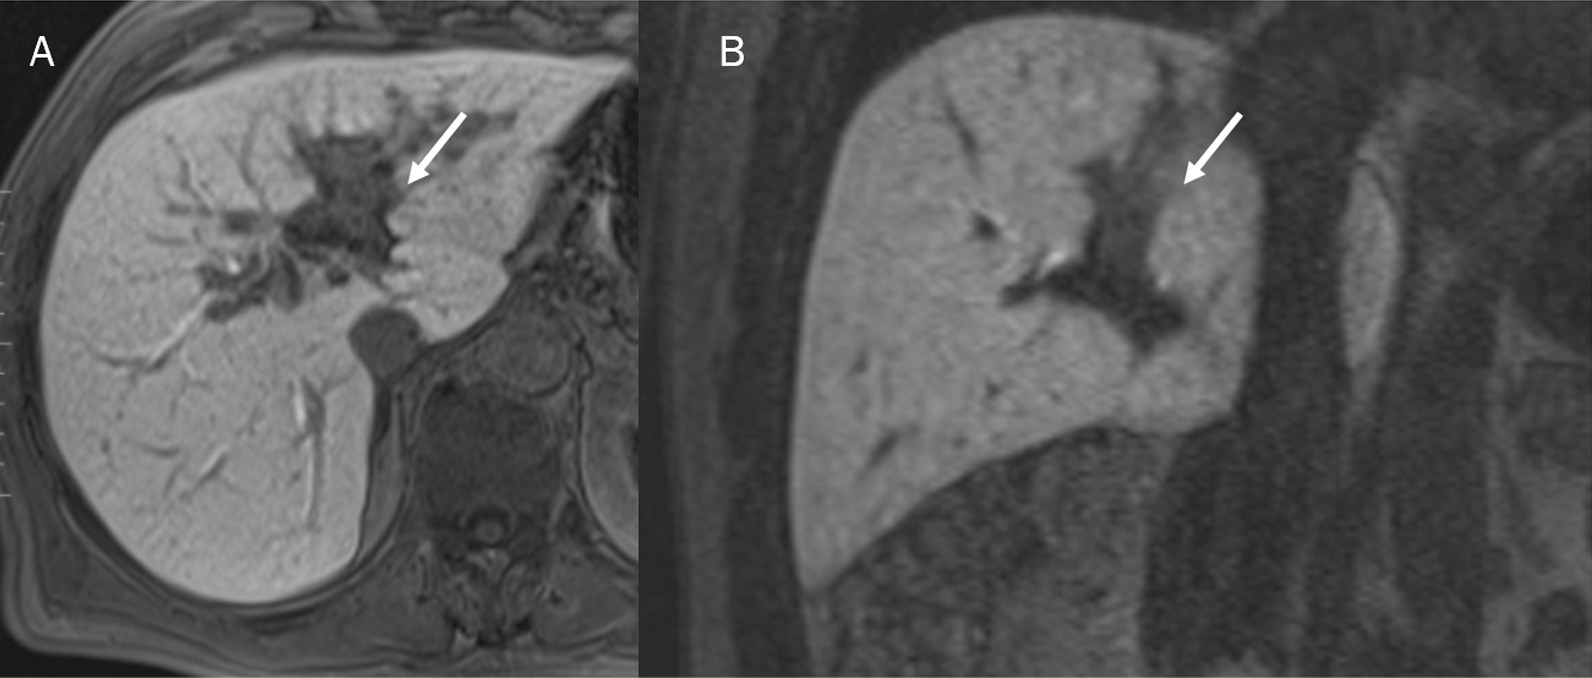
MRI assessment of ICC in hepatobiliary phase of contrast study. The lesion (arrows) shows hypointense signal intensity in A (axial plane) and B (coronal plane)
